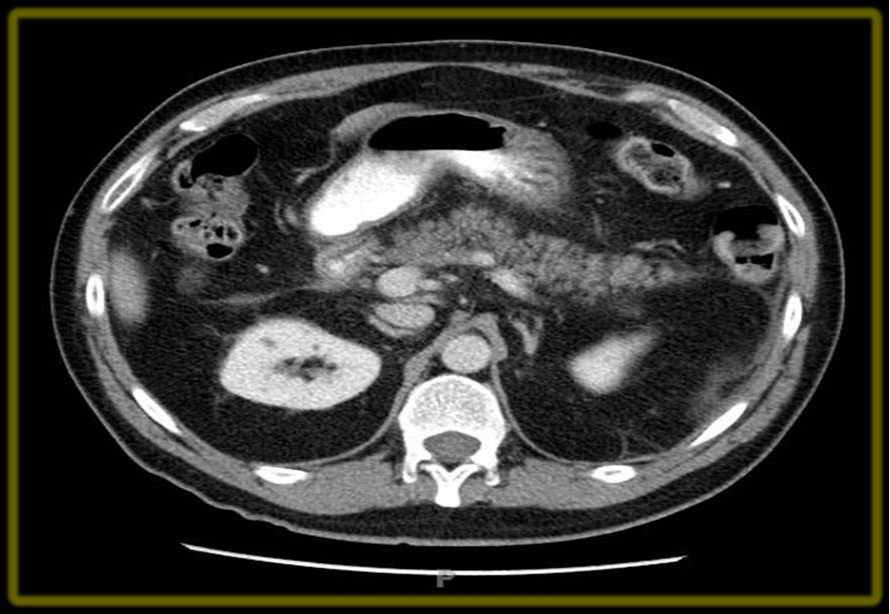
Abdominal CT scan with contrast showed an indistinct pancreatic tail with peripancreatic inflammatory changes consistent with pancreatitis.
